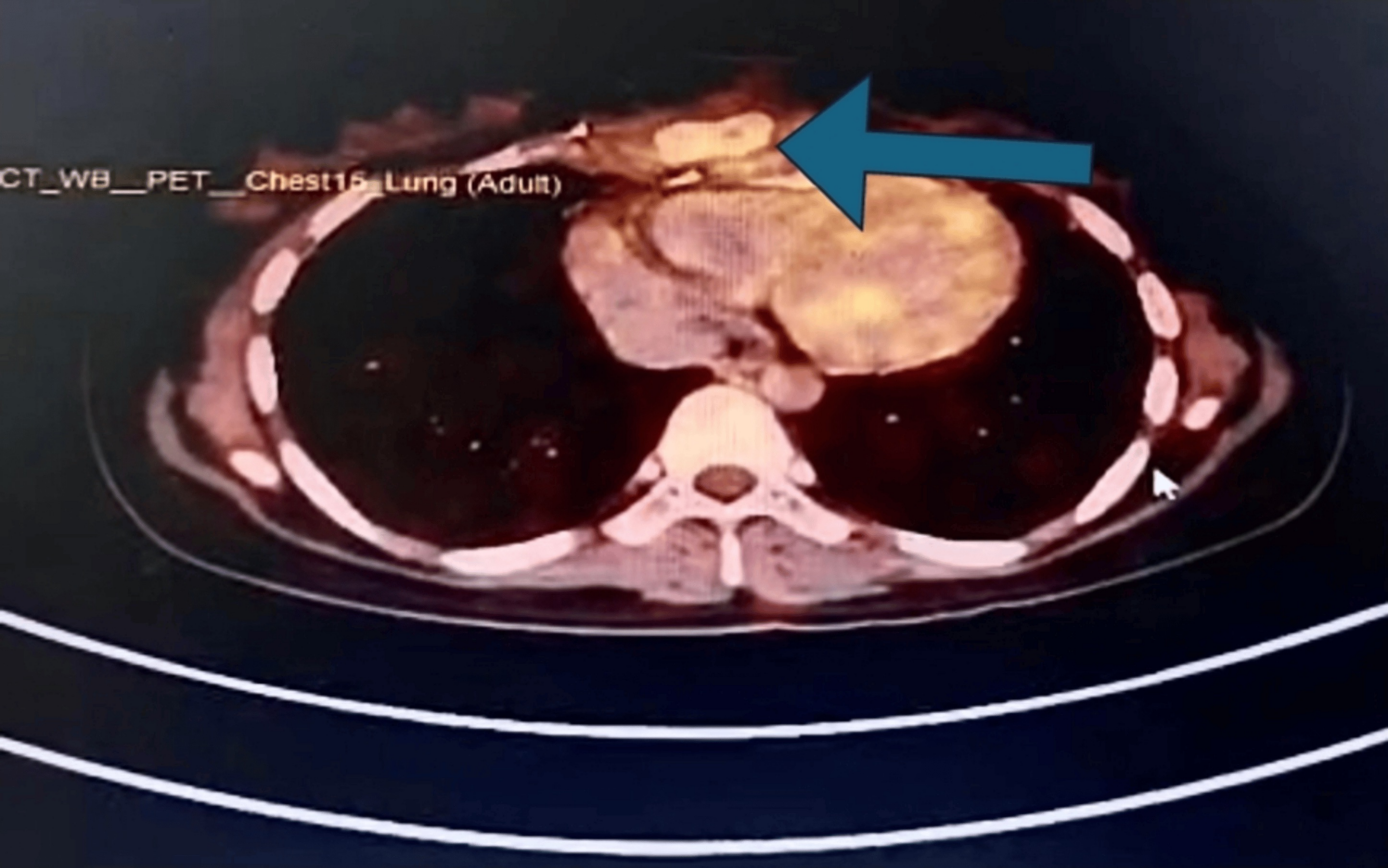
Axial PET-CT fusion image showing FDG-avid lesion in the mid-sternal region The image reveals a hypermetabolic, FDG-avid lesion involving the anterior mid-sternum, consistent with high-grade pleomorphic sarcoma. No evidence of intrathoracic or distant metastatic spread is seen. PET-CT: positron emission tomography-computed tomography, FDG: fluorodeoxyglucose
